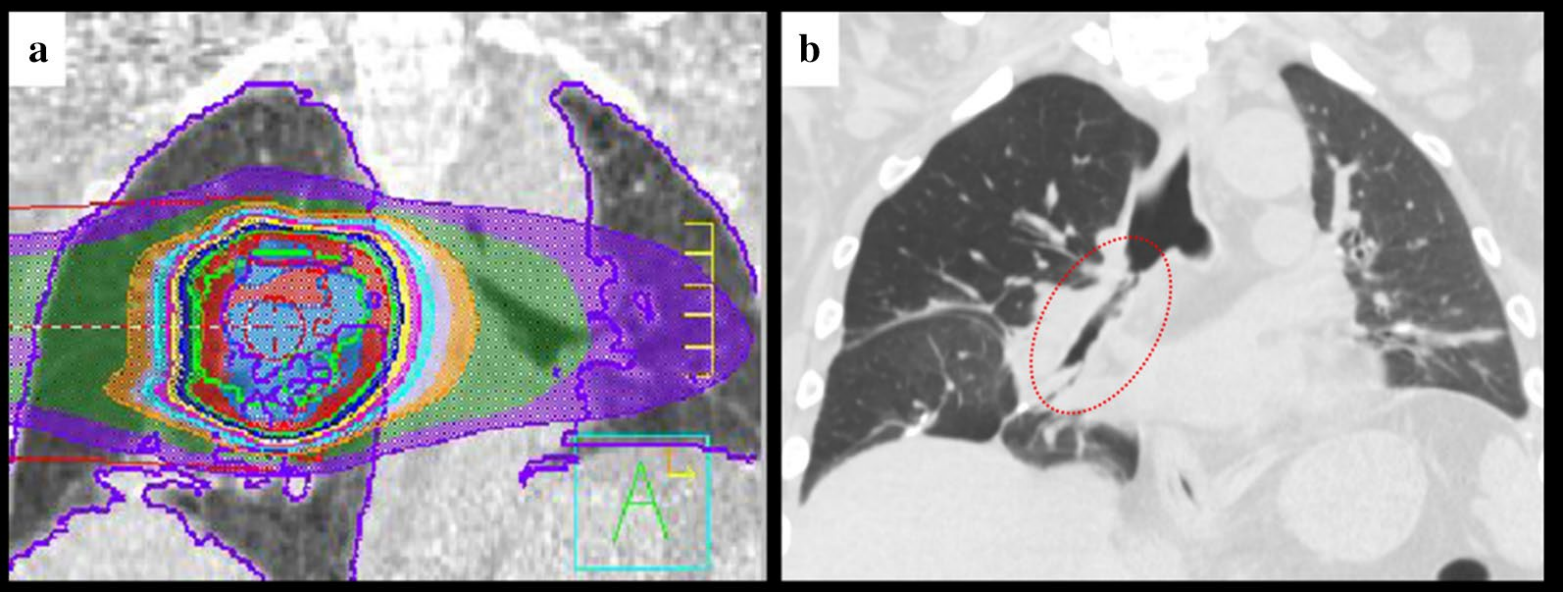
Coronal images of computed tomography before and after radiotherapy. a Radiation range shown with a coronal plane. b A coronal view showing the wide range of bronchial stenosis, indicating right bronchial wall thickening and narrowing of the bronchial lumen (red interrupted line)
